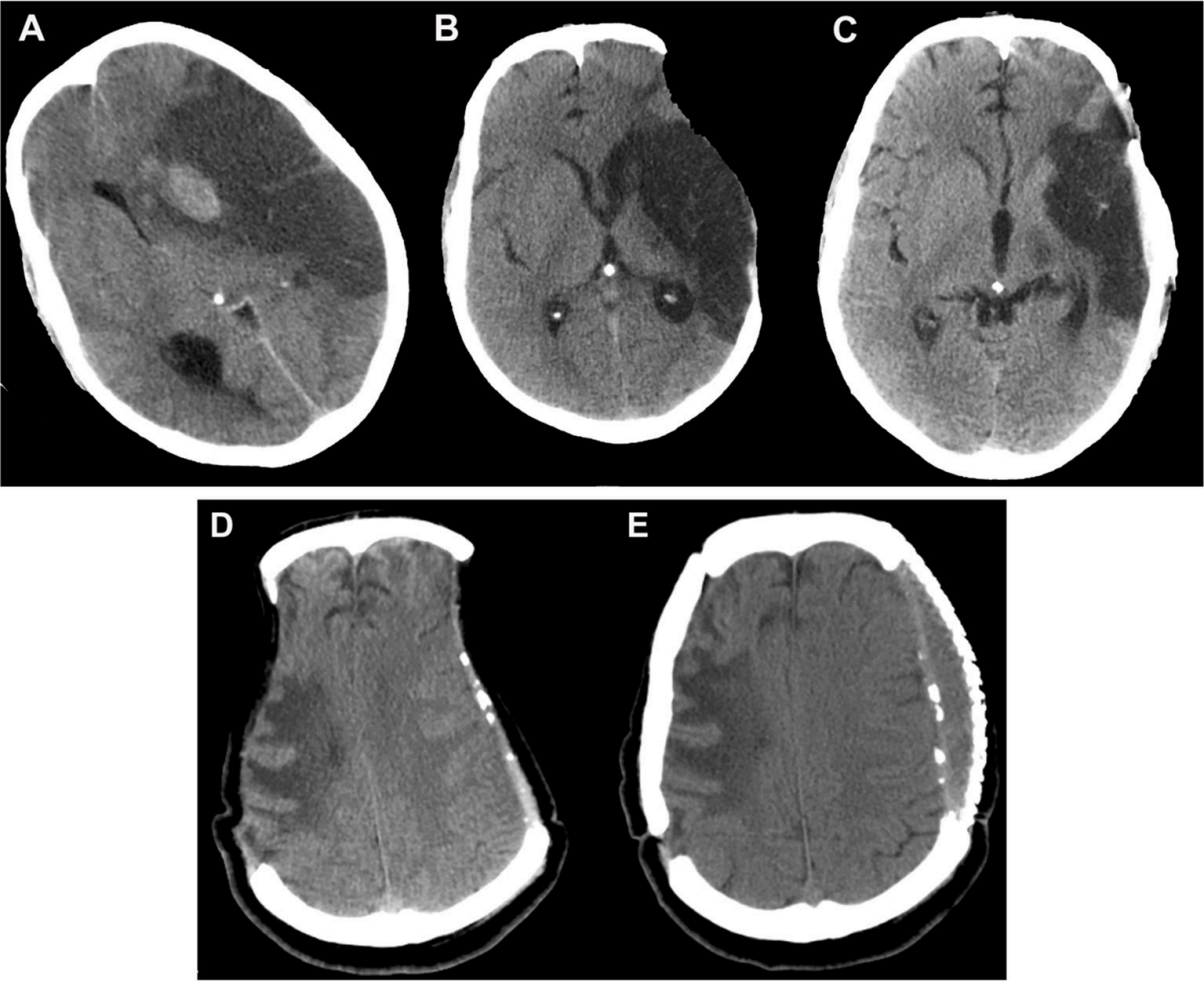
Sunken flap syndrome. Computed tomography depicting (A) malignant hemispheric infarction, (B) sunken flap syndrome after 6 months, which improved (C) post cranioplasty. In a different patient (D), bilateral sunken flap syndrome was observed 25 months post DC, and (E) improved after cranioplasty. DC, decompressive craniectomy.
